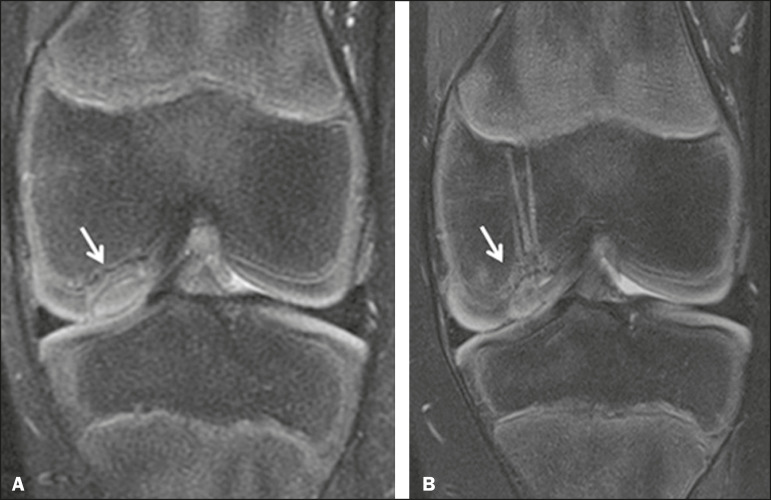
A 10-year-old boy submitted to surgical fixation of the chondral fragment. Coronal T2-weighted fat-saturated MRI sequences. Preoperative sequence (A) showing osteochondral involvement accompanied by bone marrow edema, without detachments in the central part of the weight-bearing area of the medial femoral condyle. Sequence obtained eight months after the procedure (B), showing a linear area of high signal intensity (arrow) at the subchondral cartilage/ bone interface in the inner portion of the lesion focus, representing granulation tissue. A linear area of low signal intensity at the most anterior margin of the cartilage in the focus of osteochondral injury, the site of a previous fissure, inferring coaptation, possibly with fibrocartilage tissue.
